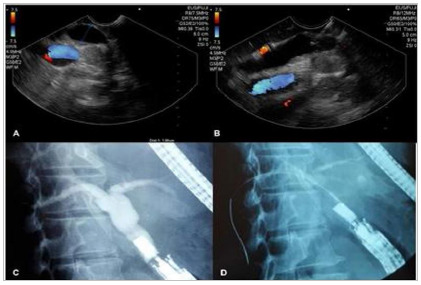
EUS-CDT. A) endosonographic identification of the common bile duct from
